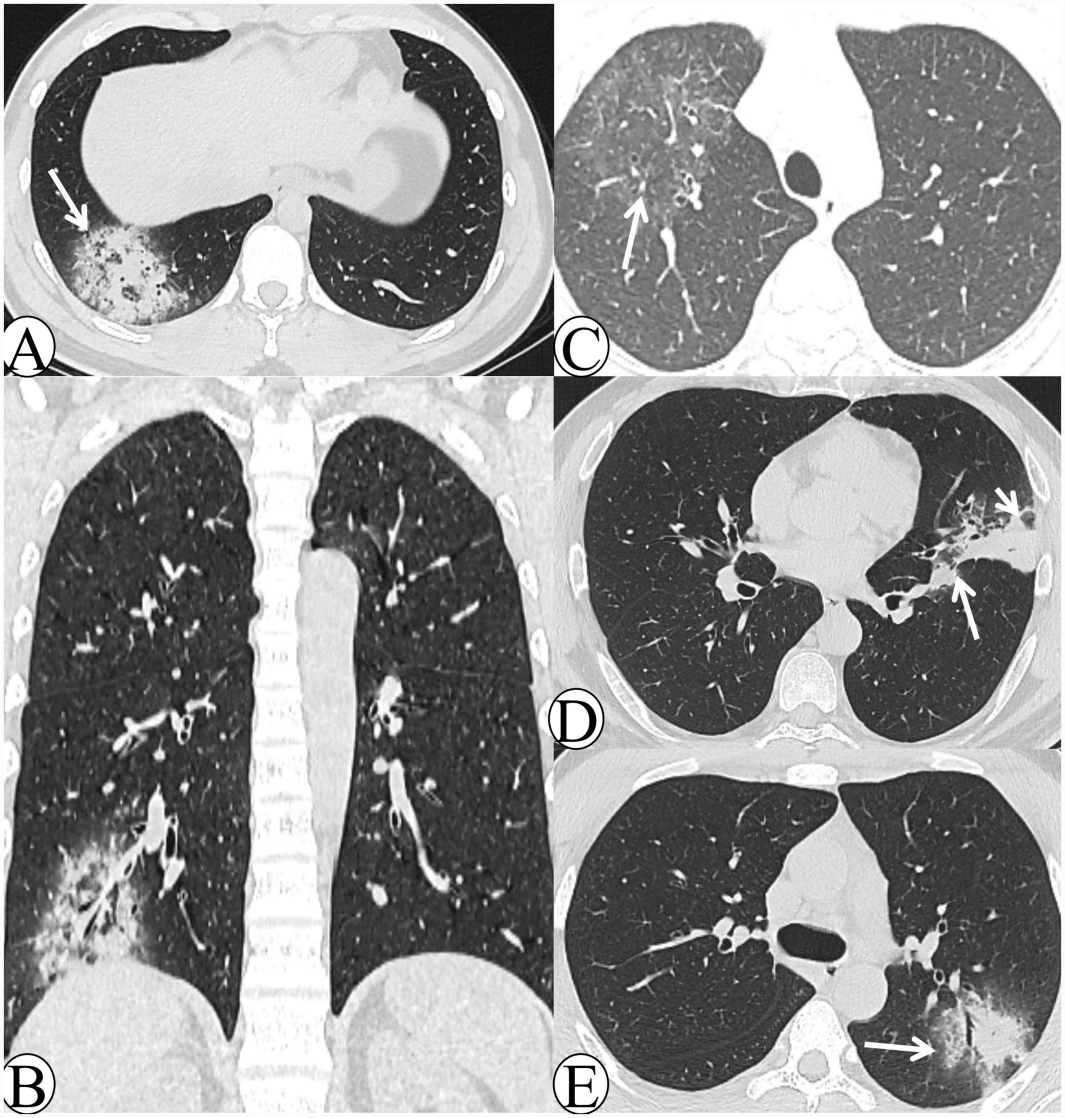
The CT images of the middle–late-stage *Chlamydia pneumoniae* pneumonia. (A) A 29-year-old male patient presented with cough and expectoration for 14 days. Axial lung window CT demonstrates mass-like consolidation in the right lower lobe with peripheral halo sign changes, and multiple reversed tree-in-bud signs are visible within the lesion (white arrow). (B) The same patient in image A. Coronal lung window CT demonstrates that the lesion exhibits a reversed halo sign appearance. (C) A 16-year-old male patient presented with cough and expectoration for 5 days. Follow-up axial lung window CT after 14 days of treatment demonstrates significant resolution of the lesion, which exhibits a faint reversed halo sign (white arrow). (D) A 47-year-old male patient presented with cough and expectoration for 20 days. Axial lung window CT demonstrates patchy consolidation in the left upper lobe, with lobular sparing beneath the pleura (white arrowhead) and along the interlobar fissure (white arrow). (E) A 21-year-old male patient presented with chills and fever for 1 day. Follow-up axial lung window CT obtained after 8 days of symptomatic treatment demonstrates peribronchial patchy consolidation and ground-glass opacity (GGO) in the left upper lobe, with visible intralobular septal thickening (white arrow).
